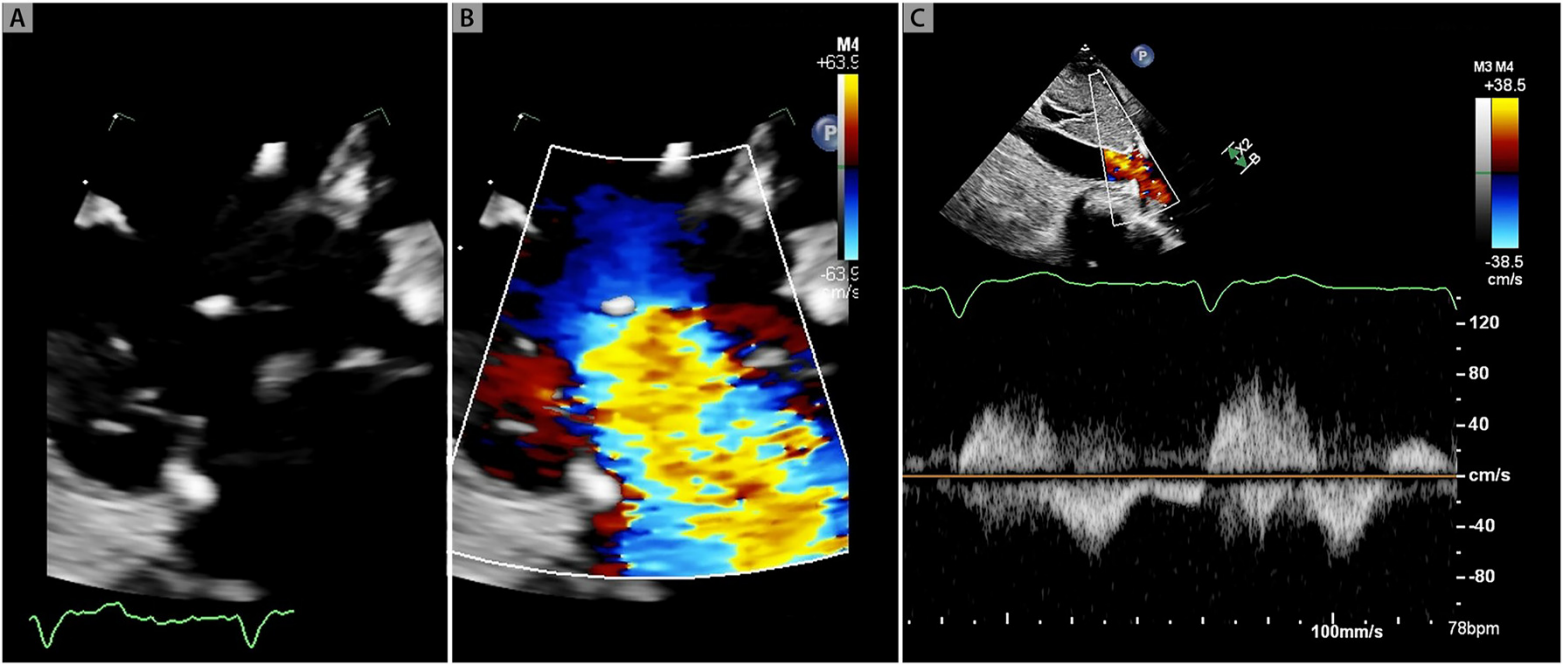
Echocardiogram images showing severe TR. Right ventricular inflow view. Malcoaptation of the tricuspid valve leaflets (panel A). Severe tricuspid regurgitation by color Doppler (panel B). Systolic flow reversal in the hepatic vein (panel C).
